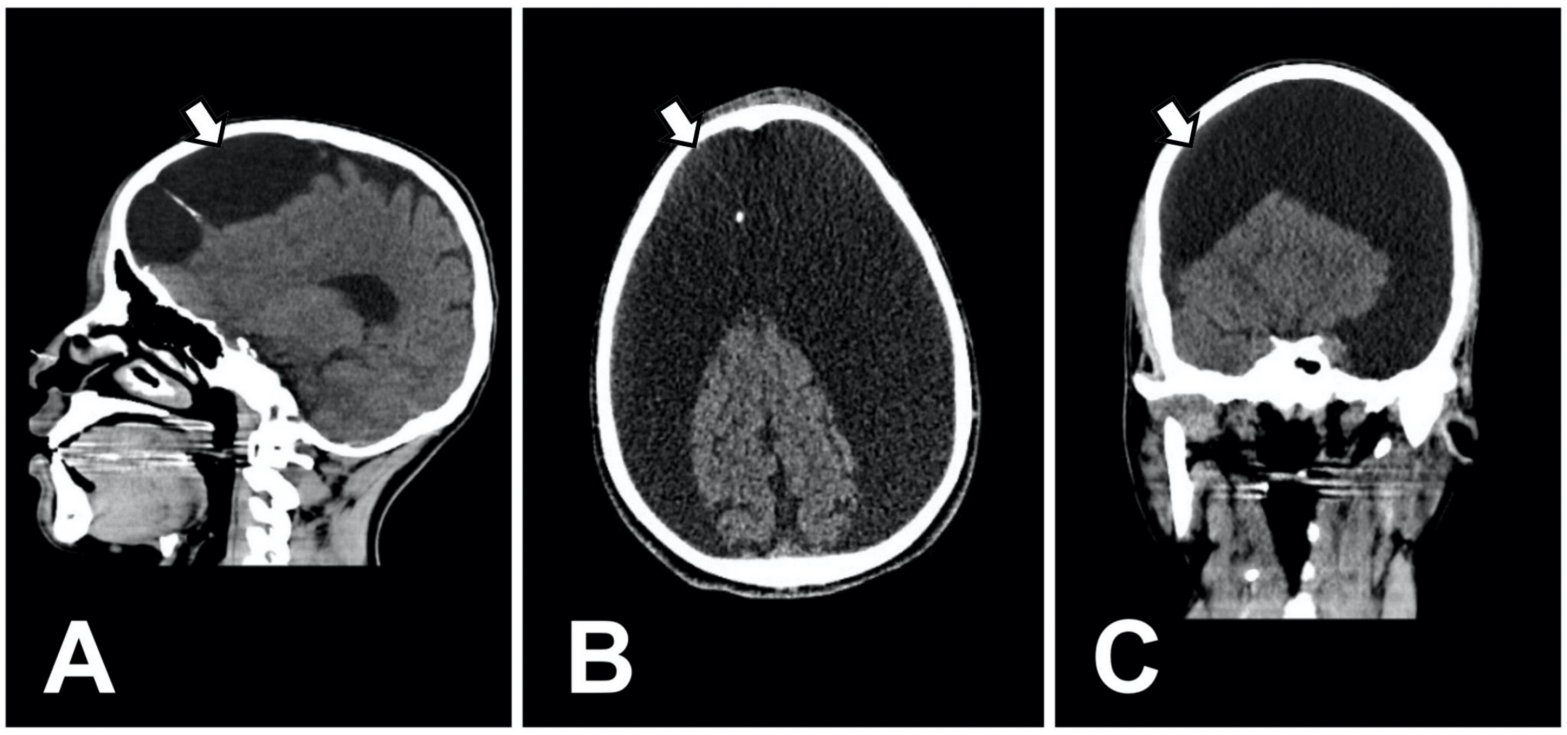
Non-contrast cranial computed tomography (CT) CT scan with sagittal (A), axial (B), coronal (C) views reveals ovoid lesions in the frontotemporal region (arachnoid cysts). No abnormalities are observed in the brainstem or cerebellum, and normal differentiation between gray and white matter is maintained.
